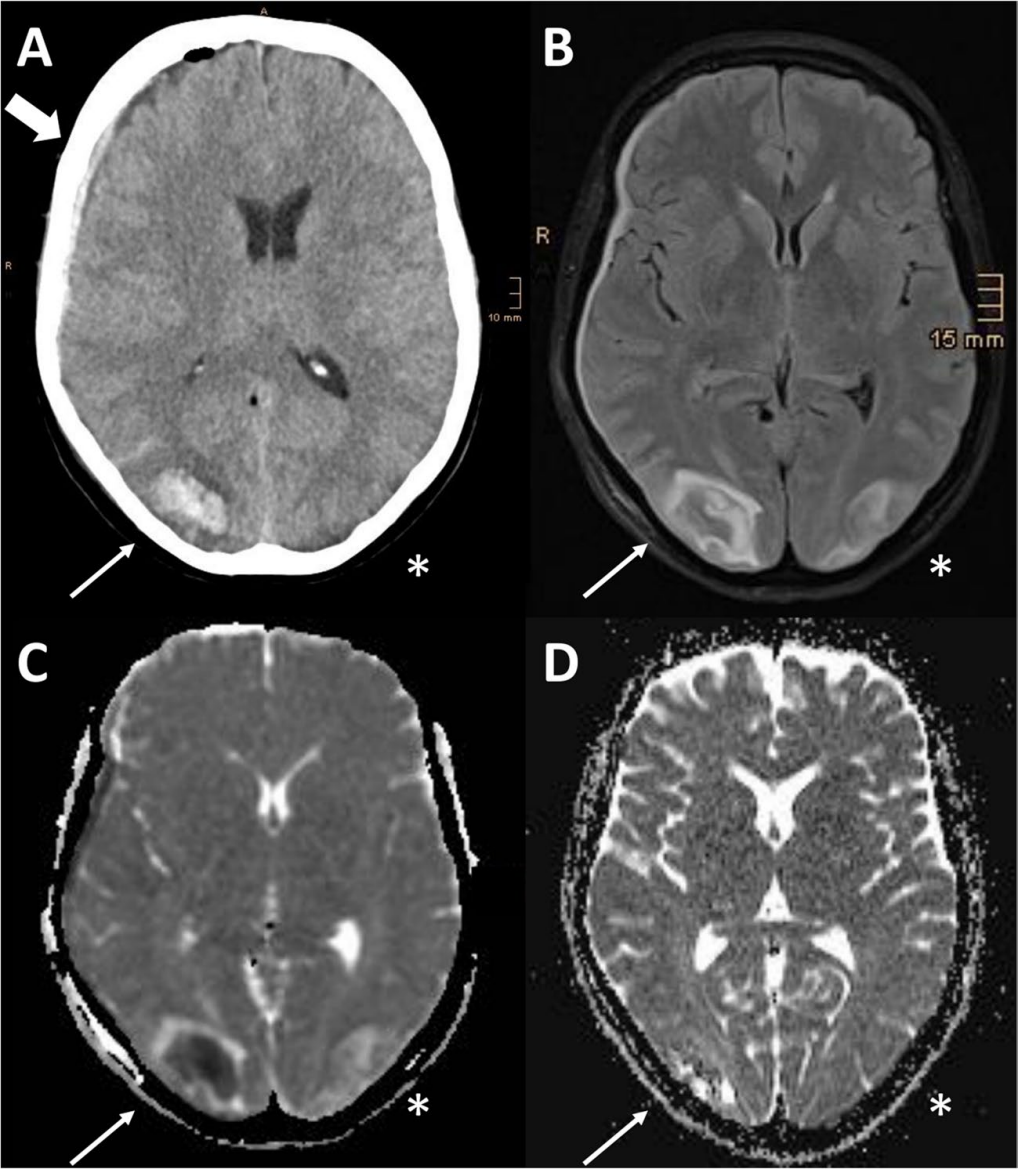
Imaging findings in our case of PRES after metronidazole administration. Native brain CT imaging showed A) right-sided occipital intracerebral haemorrhage with peripheral oedema (thin arrow), subdural bleeding (large arrow); left-sided grey-white matter junction oedema (*). MRI performed 2 days after clinical onset revealed bilateral occipital B) FLAIR (thin arrow and *) and C) ADC hyperintensities (thin arrow and *) indicating vasogenic edema. The relative right occipital hypointensity on the ADC map is related to the haemorrhage. D) Follow-up MRI after 2 months from clinical onset showed in the ADC map almost complete resolution of the vasogenic oedema and subtotal resorption of parenchymal haemorrhage (thin arrow and *). Abbrevations. ADC: apparent diffusion coefficient; CT: computed tomography; FLAIR: fluid-attenuated inversion recovery; MRI: magnetic resonance imaging; PRES: posterior reversible encephalopathy syndrome
